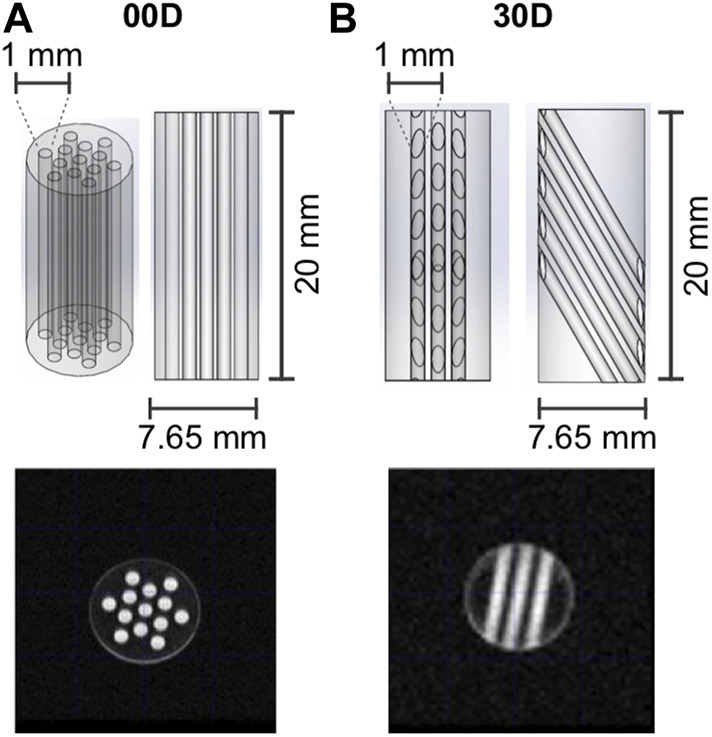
Geometry and dimensions of channel structures (A) 00D and (B) 30D, which were used in the experiments reported in this paper. The MRI images were generated with a multi-slice multi-echo (MSME) sequence (FOV 2 × 2 cm^2^, 128 × 128 matrix, slice thickness 1 cm, TE = 5.08 ms). Sagittal slices (not shown) were taken to confirm that all channels were fully filled with the EPM solution.
